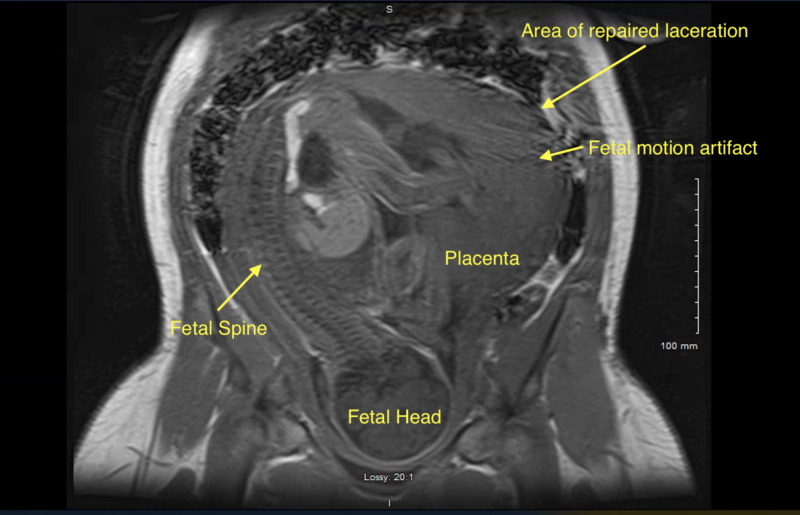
MRI at 31 4/7 weeks with motion artifact due to fetal movement, without evidence of uterine rupture.
